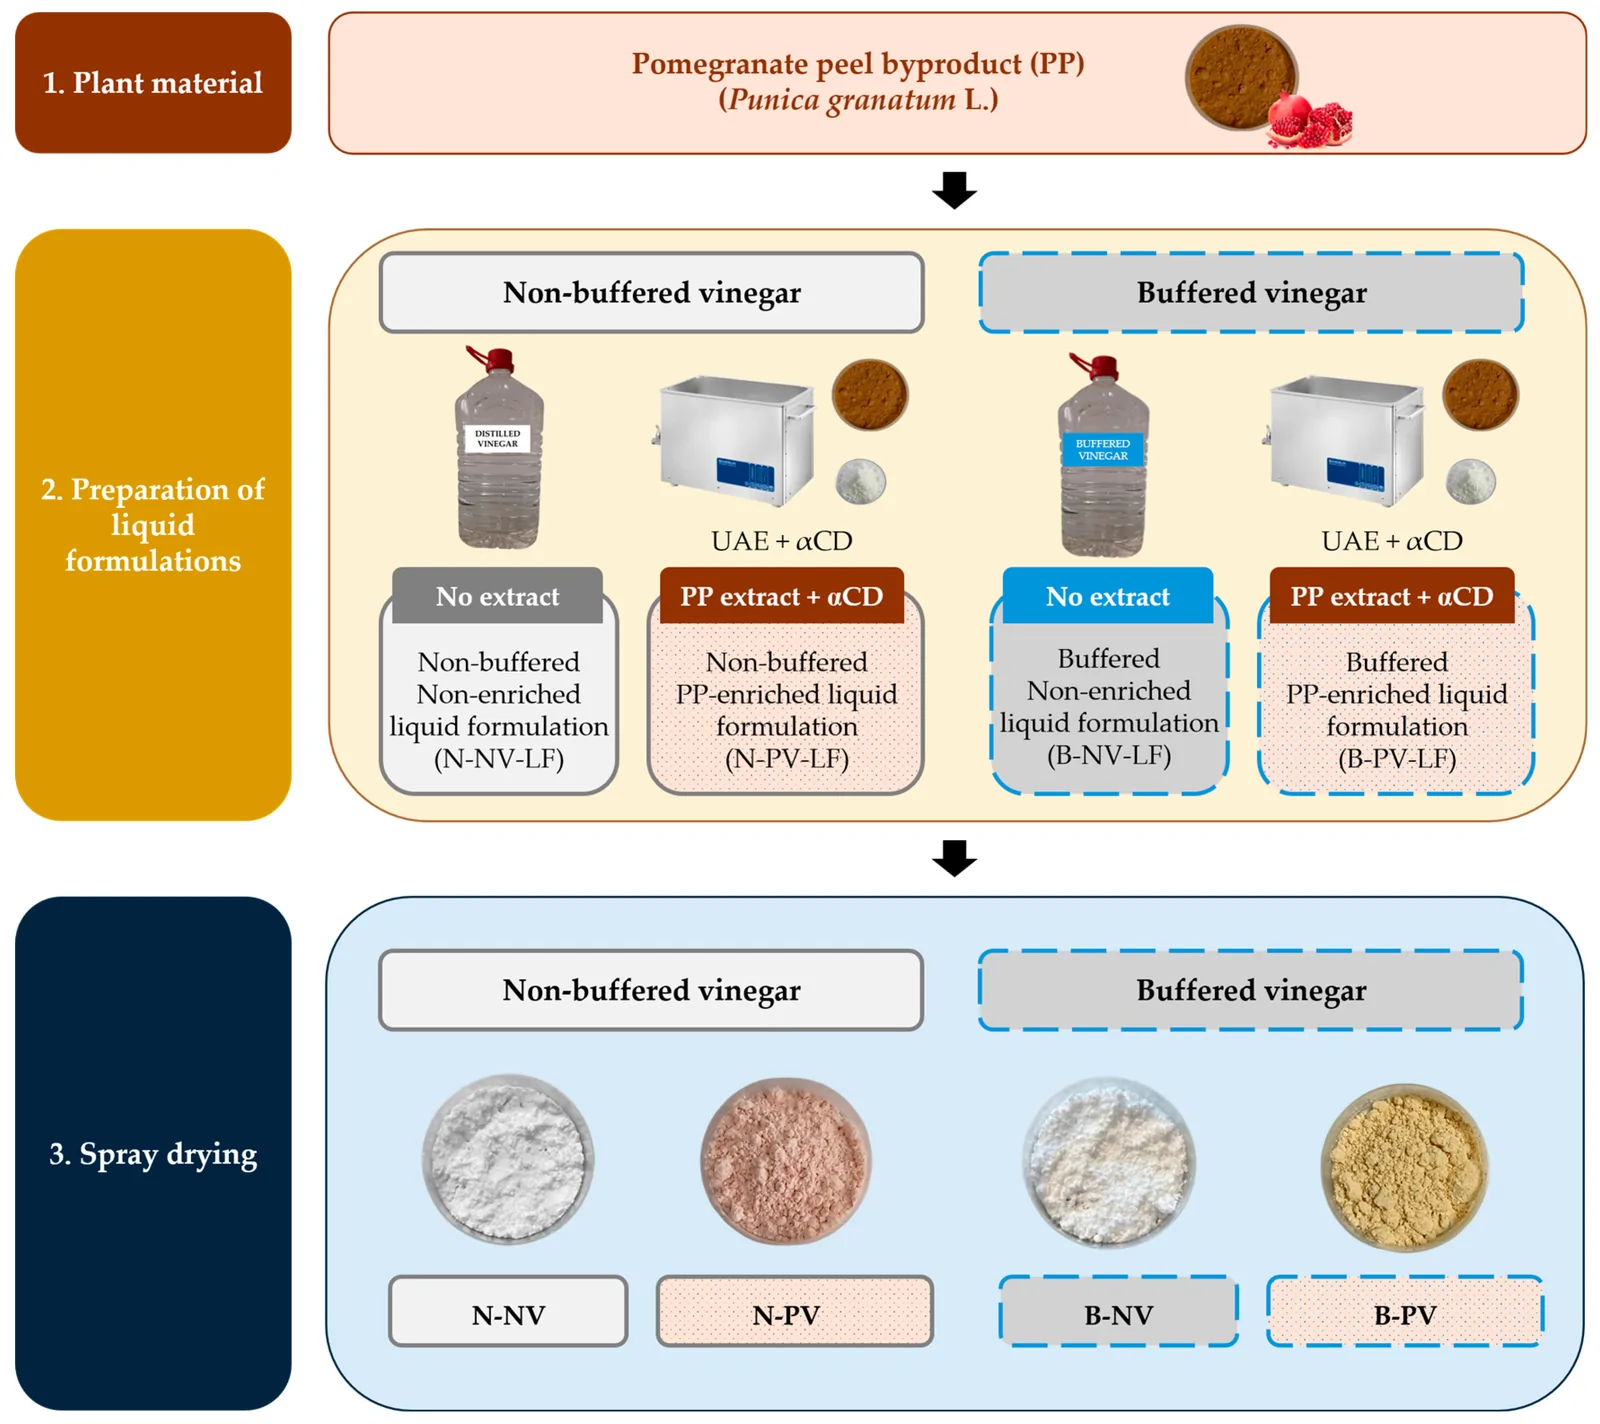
Experimental workflow for the preparation and spray drying of non-enriched (N-NV and B-NV) and pomegranate peel extract-enriched (N-PV and B-PV) powder formulations. PP = pomegranate peel byproduct; UAE = ultrasound-assisted extraction; αCD = α-cyclodextrin; LF = liquid formulation; N-NV = non-buffered non-enriched vinegar powder; N-PV = non-buffered pomegranate peel extract-enriched vinegar powder; B-NV = buffered non-enriched vinegar powder; B-PV = buffered pomegranate peel extract-enriched vinegar powder.
